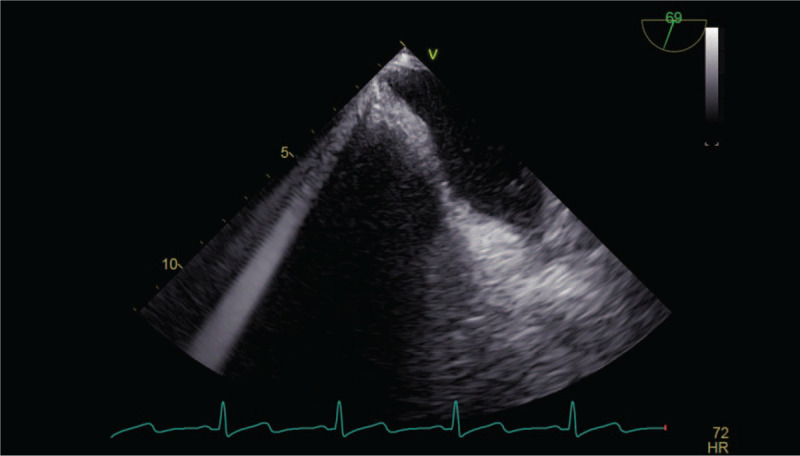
TEE of clamping of the inferior vena cava. TEE = transesophageal echocardiography.
